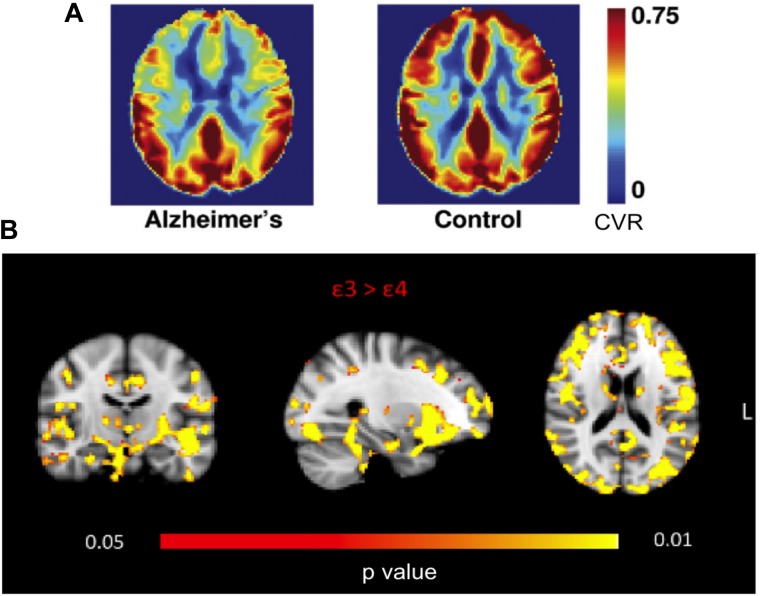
MRI-based CVR maps in AD and APOE gene carriers. (A) Frontal, cingular, and insular CVR deficits are found in AD patients. Figure is modified from Yezhuvath et al. (2012) with permission. (B) Young 𝜀4 carriers manifest widespread CVR deficits compared to 𝜀3 homozygotes. Figure is modified from Suri et al. (2014) with permission.
